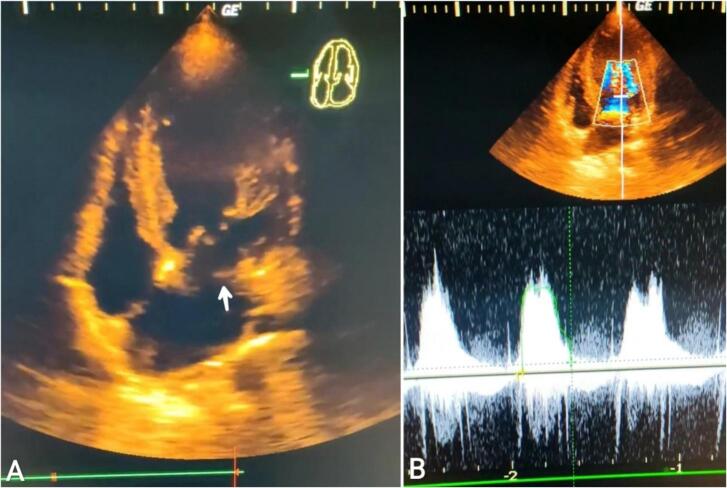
Two-dimensional (2D) transthoracic echocardiography: Image (A): Apical 4-chamber view showing membranous supramitral ring (white arrow). Image (B): Continuous wave doppler across the mitral valve demonstrates severe supravalvular mitral stenosis with a mean diastolic pressure gradient of 12 mmHg.
